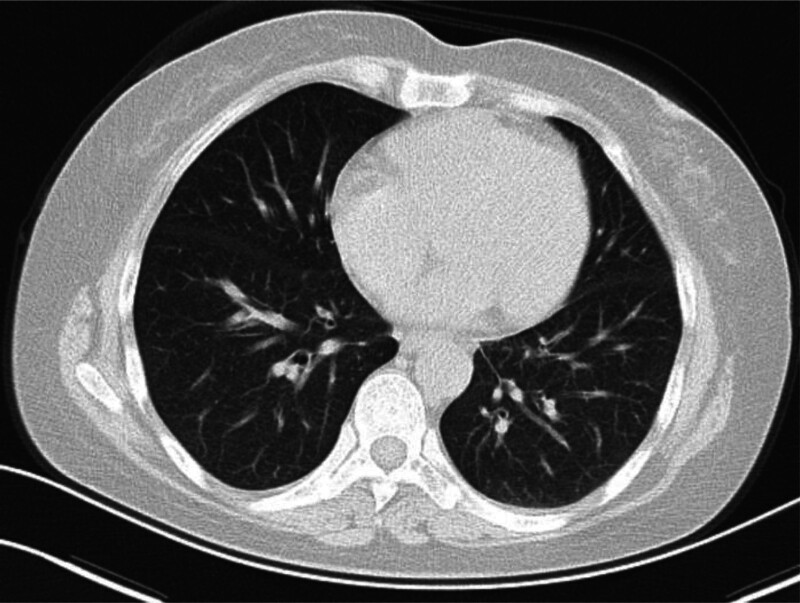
Chest computed tomography scan shows a few inflammatory and fibrous foci in both lungs and pulmonary interstitial inflammatory changes with thickening of interlobular septa.
